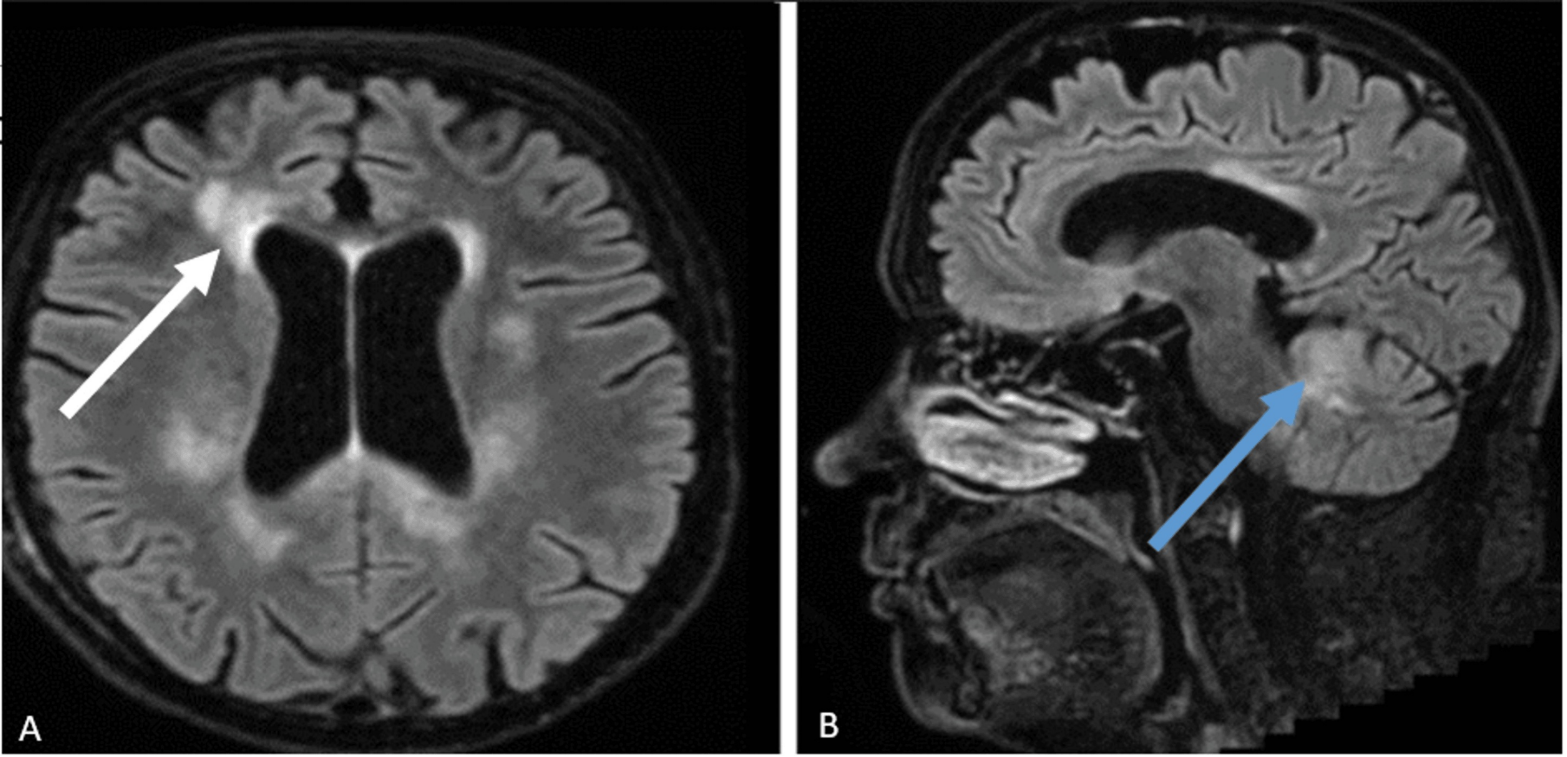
Brain MRI findings three months after corticosteroid therapy: Axial (A) and sagittal (B) FLAIR images demonstrate a hyperintense cortico-subcortical lesion in the right frontal lobe (white arrow) and a hyperintense lesion in the cerebellum (blue arrow). MRI, magnetic resonance imaging; FLAIR, fluid-attenuated inversion recovery
